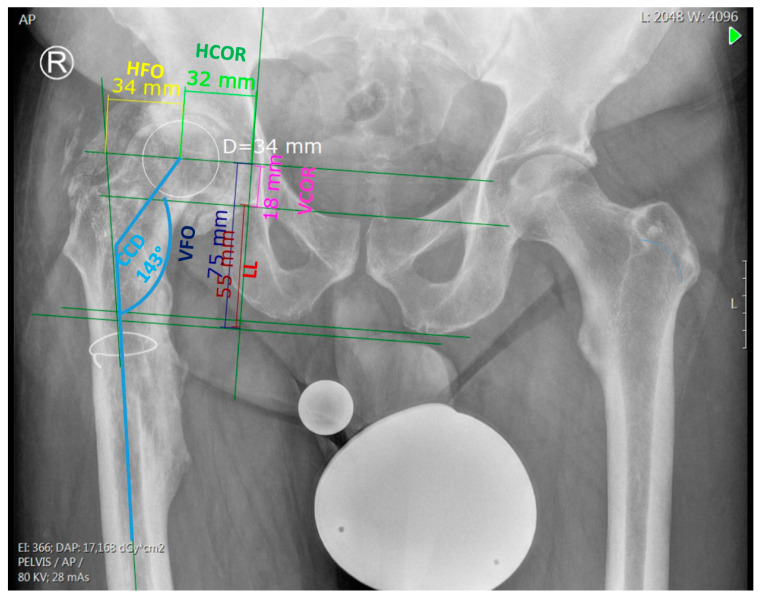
Preoperative measurements on AP pelvis X-ray. HFO: horizontal femoral offset. VFO: vertical femoral offset. HCOR: horizontal center of rotation. VCOR: vertical center of rotation. CCD: projected neck-to-shaft angle. LL: leg length.
